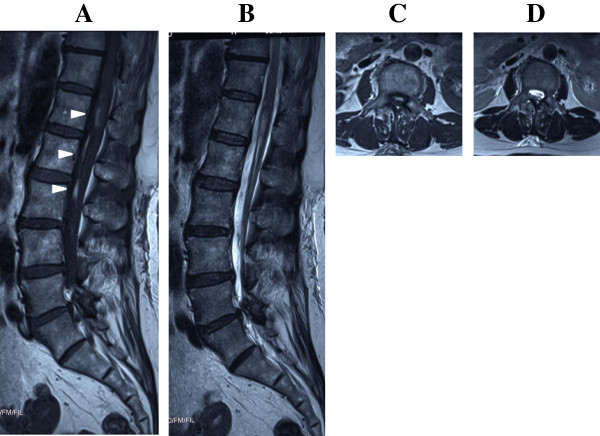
Magnetic resonance imaging 8 days after onset. Sagittal T1-weighted (A) and sagittal T2-weighted (B) images show subarachnoid hemorrhage extending from L1 to L3 ventrally to the spinal cord (arrowheads). There is no severe compression of the cord. The axial T1-weighted (C) and axial T2-weighted (D) images are at L1.
